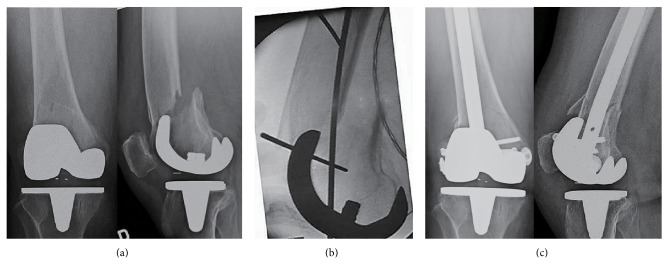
(a) Radiographs of a fracture located proximal to the right femoral component of TKA. (b) On the lateral side, 2.4 mm blocking pins inserted into the distal and proximal fragments. (c) Acceptable reduction and fixation of the fracture with a nail.
